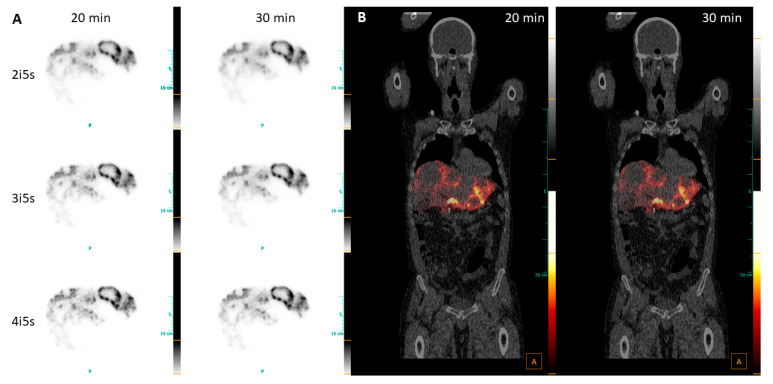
(A) Transversal views of PET images of patient 8, diagnosed with CRC reconstructed with 2i5s, 4 mm filter; (B) fused coronal PET/CT images, reconstructed with 2i5s, 4 mm filter.
